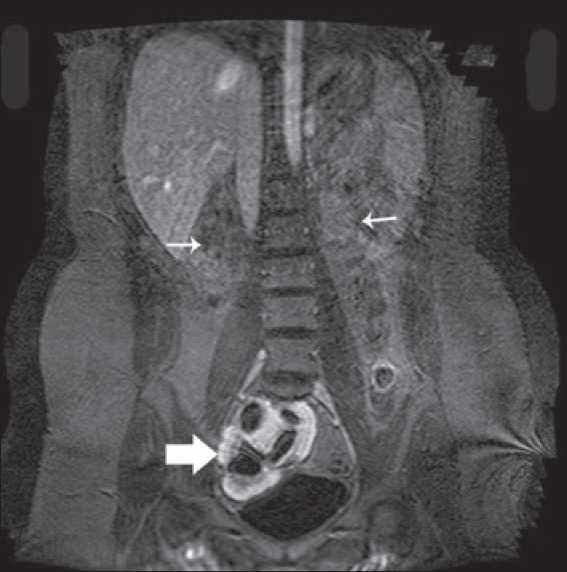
MRI showing empty renal fossae (thin arrow) and pelvic kidney in relation to bladder (thick arrow)
